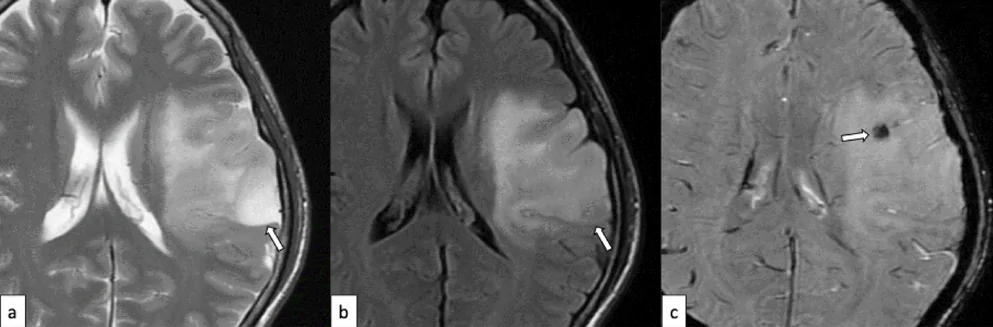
Astrocytoma, IDH-mutant, CNS WHO grade 4 in a 55-year-old woman. The tumor mass shows hyperintensity on T2-weighted image (a), both hyperintensity and iso–hypointensity on fluid-attenuated inversion recovery (FLAIR) image (b), with evidence of intratumoral hemorrhage on susceptibility-weighted image (c, arrow). Partial T2-FLAIR mismatch sign is positive in this case (a, b, arrows)
